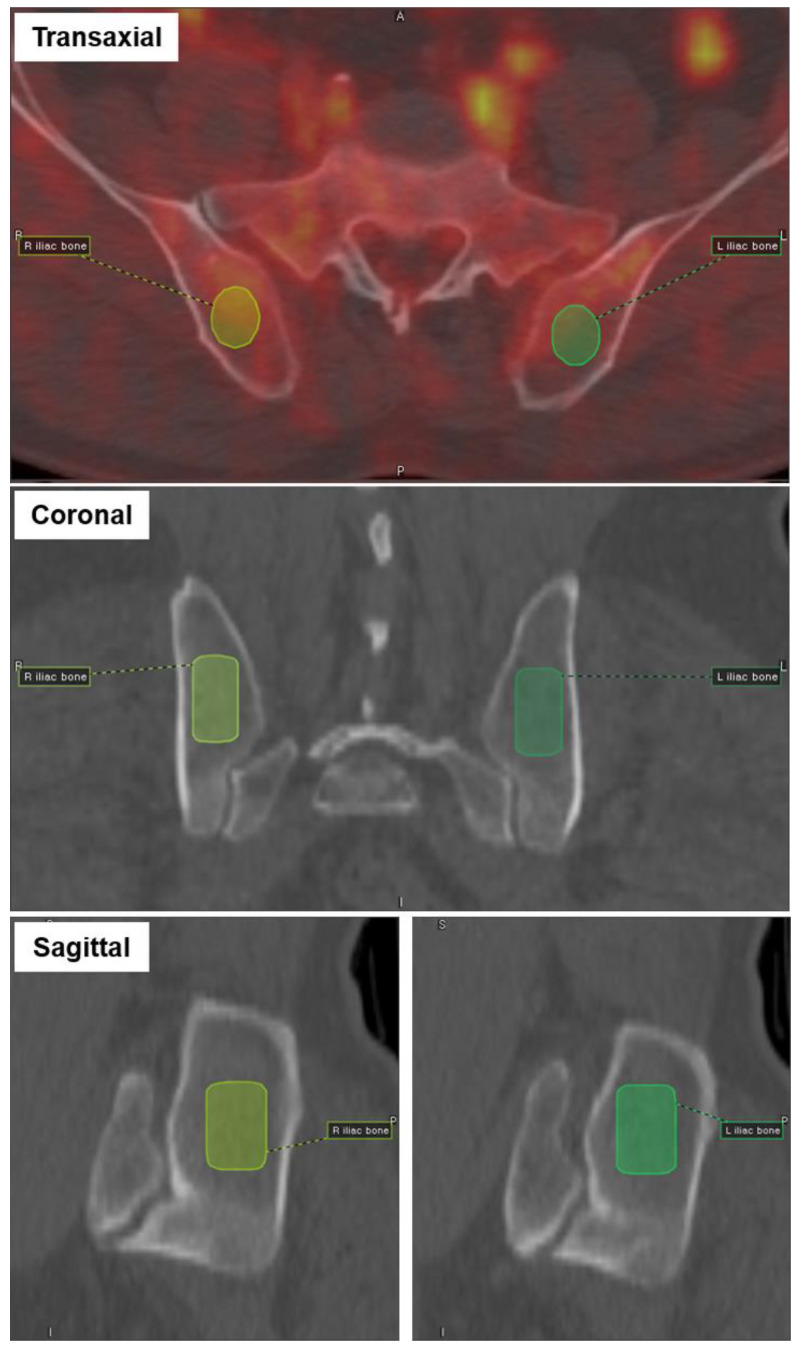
Cylinder volume of interests (VOIs) were placed on bilateral posterior iliac bones, and the CT images were reviewed to ensure the cortex of the bone was not included. The process was repeated to draw VOIs in bilateral femurs.
